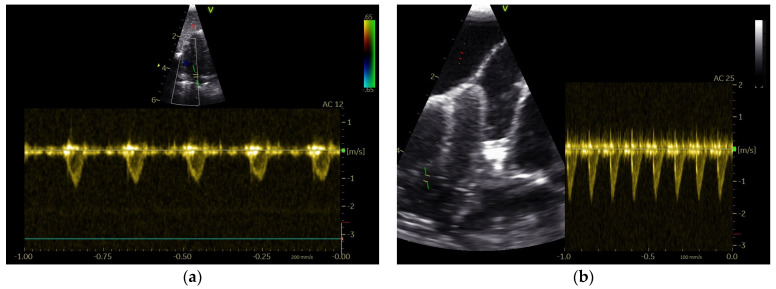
PW Doppler sonographic examination of the pulmonary blood flow profile of two Congo Grey parrots: (a) PAFP of a healthy Congo Grey parrot with a long AP and short DP; (b) PAFP of a Congo Grey parrot with left and right heart failure; the shape is similar to that of the AOFP, with a short AP and a long DP.
